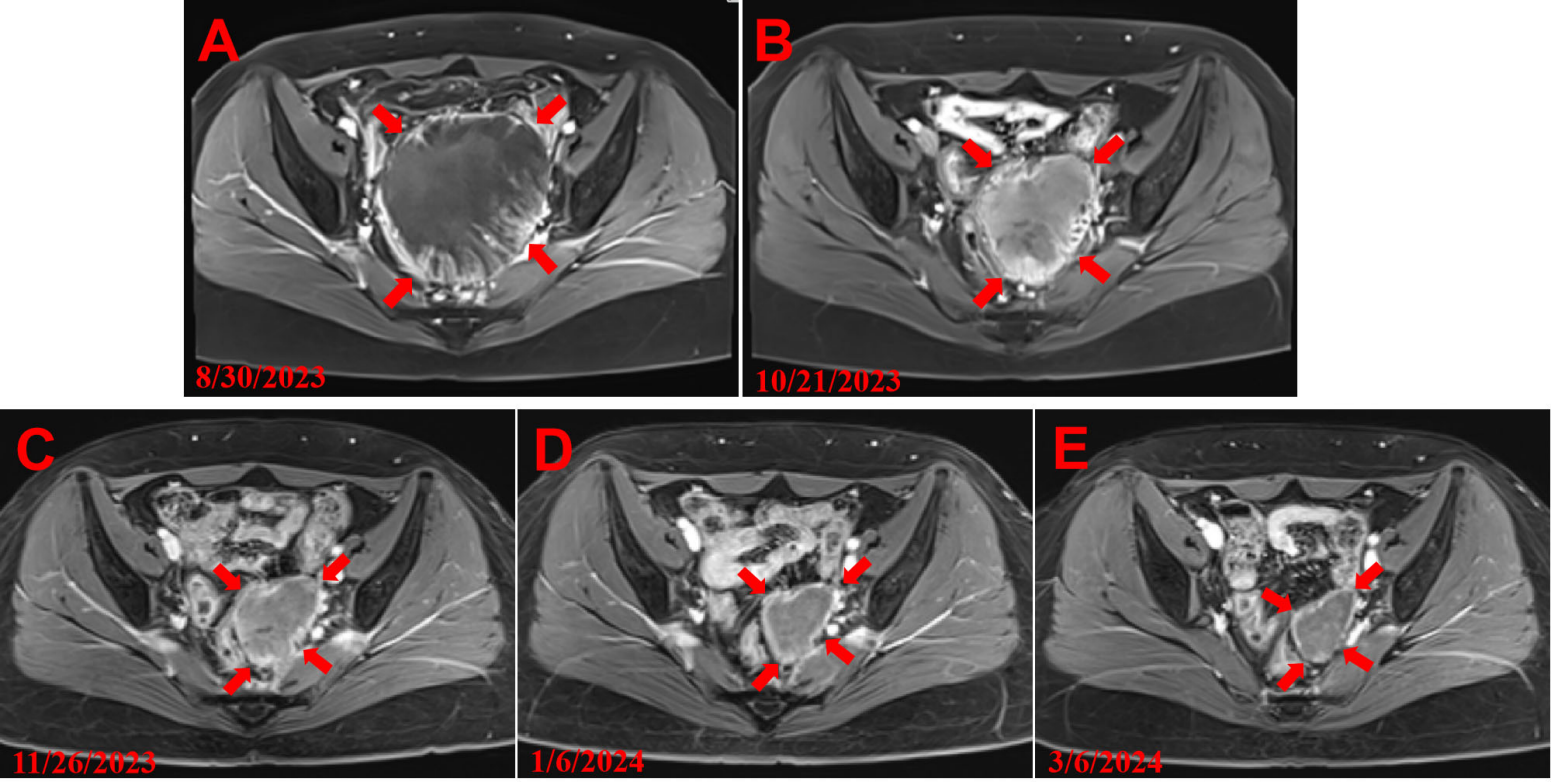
MRI images of the patient. (A) Tumor volume size of the patient’s first MRI after recurrence. (B) Tumor volume size before the first HIFU treatment after three courses of chemotherapy with TC regimen. (C) Tumor volume size after first HIFU treatment. (D) Tumor volume size after the fourth course of TC regimen chemotherapy. (E) Tumor volume size after the second HIFU treatment.
